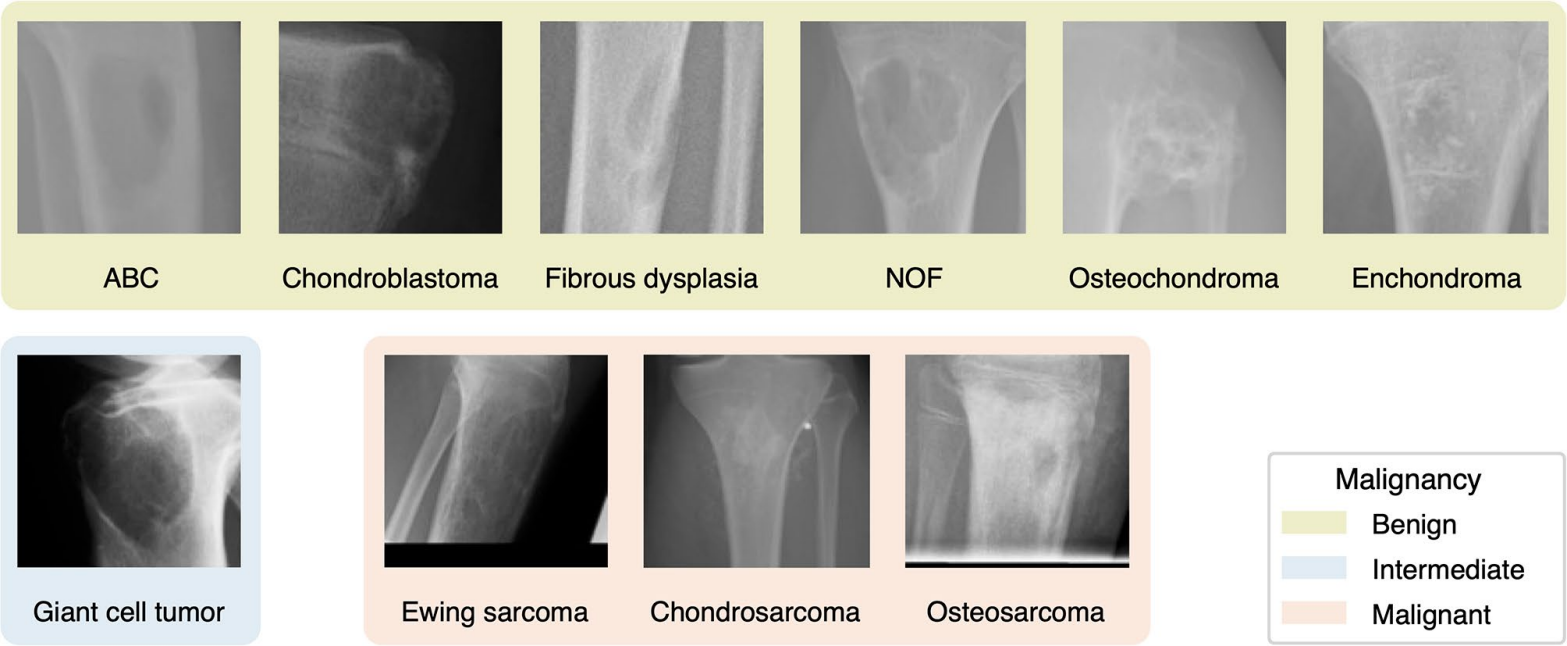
Examples of radiographs of each entity: one example for each entity after pre-processing (cropping, rescaling)
